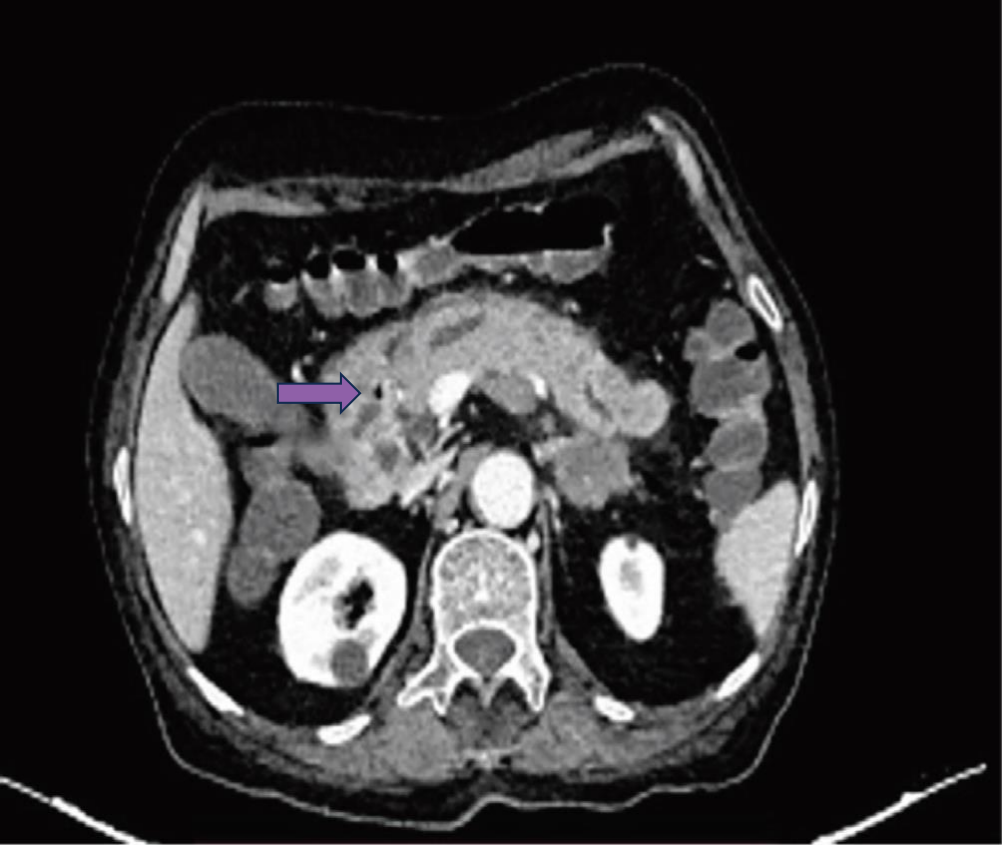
Contrast‐enhanced computed tomography of the abdomen showing acute interstitial pancreatitis (arrow) with multiple mesenteric, peripancreatic, gastro‐hepatic, and retroperitoneal lymph nodes.
